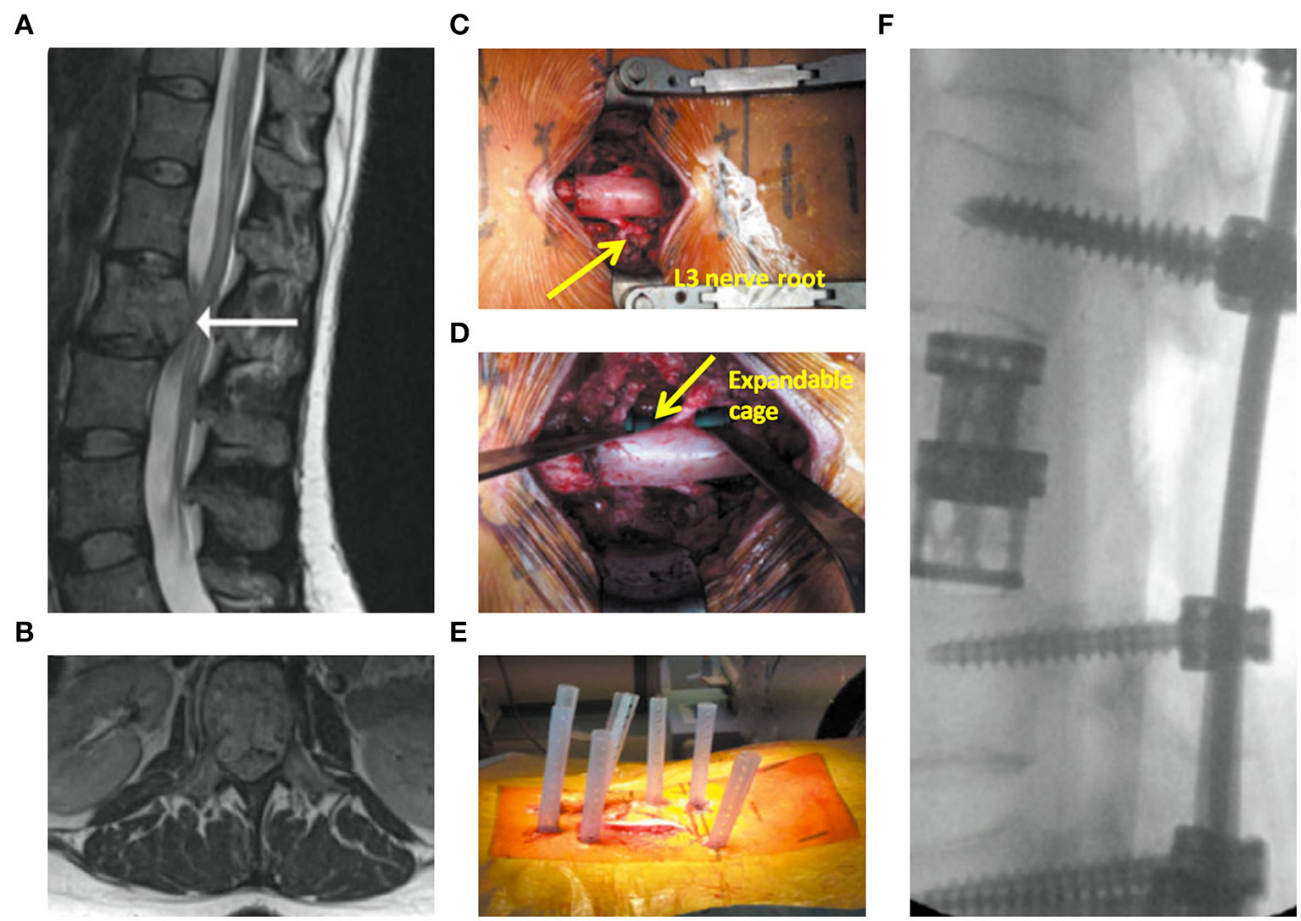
Expandable cage-assisted treatment (39). A 32-year-old female presented with mid-back pain and leg weakness >3 weeks. (A,B) Magnetic resonance imaging images showing a solitary L2 lesion causing compression of the circumferential cauda equina. (C) Intraoperative photograph showing a mid-line incision with circumferential decompression and vertebrectomy. Decompressed L3 nerve roots are displayed (arrow). (D) Intraoperative photograph showing expandable cage (arrow)-assisted reconstruction of the vertebral body. (E) Intraoperative photograph showing a mid-line wound closure and percutaneous fixation two levels above and below the vertebrectomy. (F) Postoperative radiograph.
